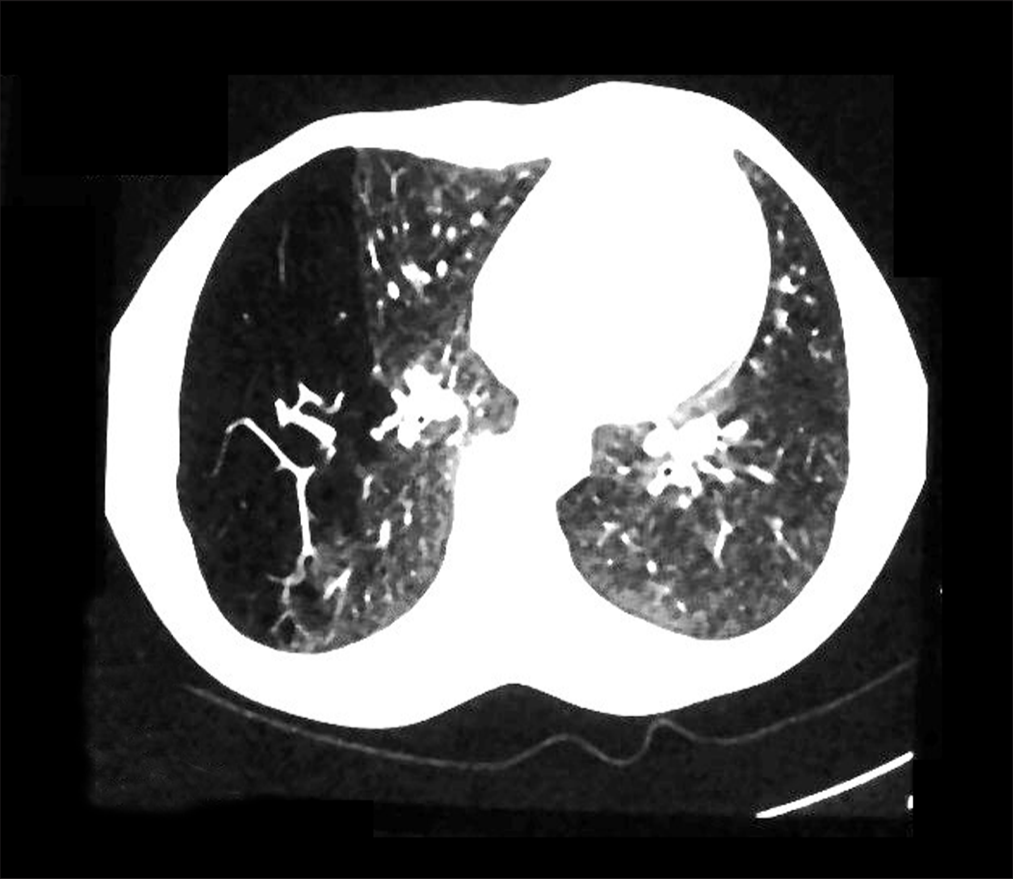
CT of chest showing right upper lobe bronchus traversing the hyperlucent lung parenchyma
